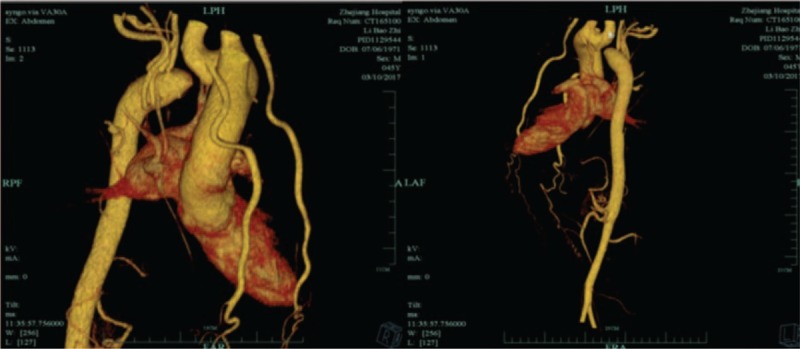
Computed tomography angiogram (CTA) revealed a type A interrupted aortic arch with complete discontinuity of the aortic lumen distal to the origin of the left subclavian artery, and a massive aorta arch which included 2 full-fledged collaterals network ensuring blood circulation to distal aorta.
